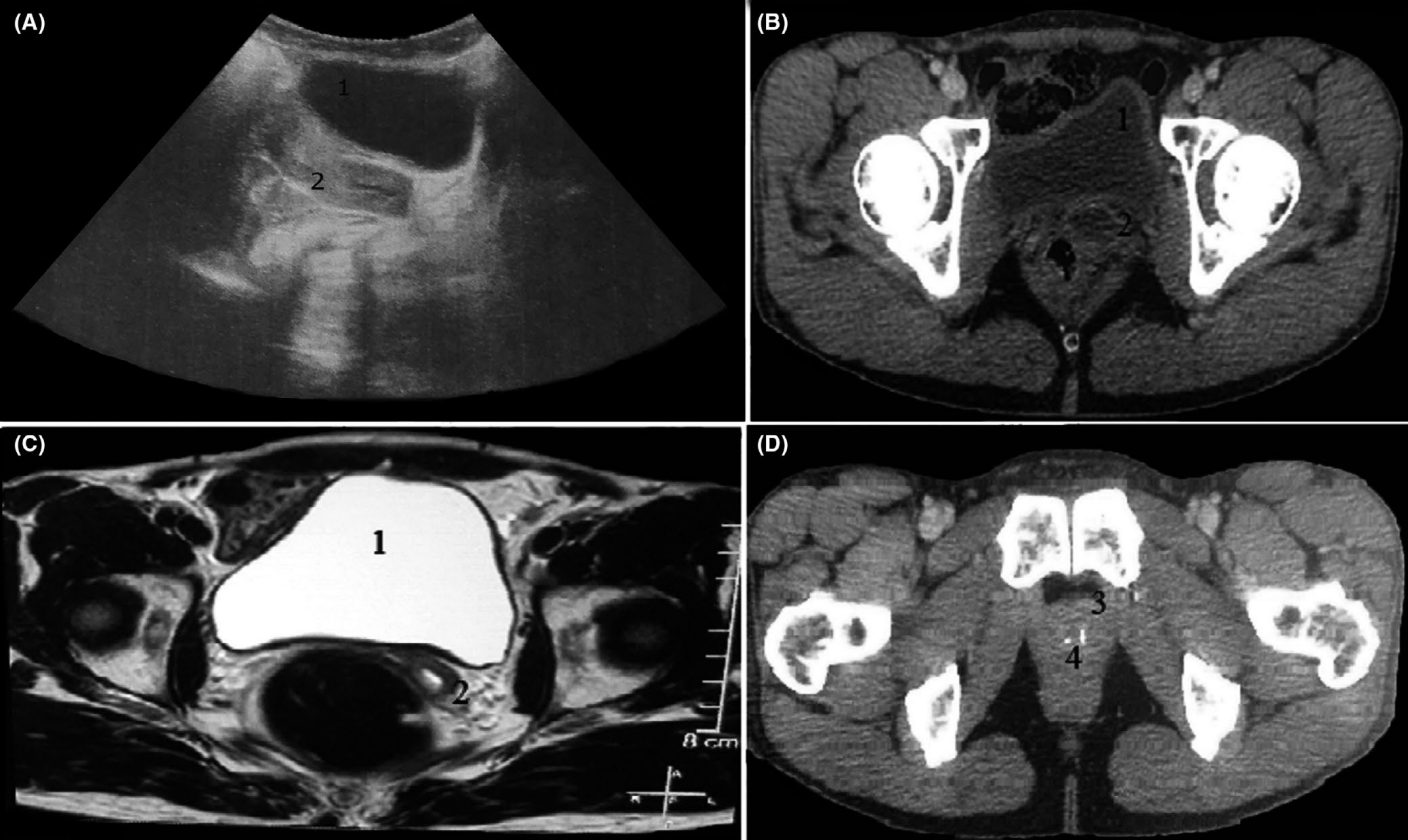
Pelvic imaging showing uterus and prostatic tissue in a 46XX patients with congenital adrenal hyperplasia due to 21‐hydroxylase deficiency. Urinary bladder (1) and uterus (2) can be seen on pelvic ultrasound (A), CT scan (B), and axial T2‐weighted MRI (C). Pelvic CT scan (D) showed also a prostatic tissue (3) containing two calcifications (4)
